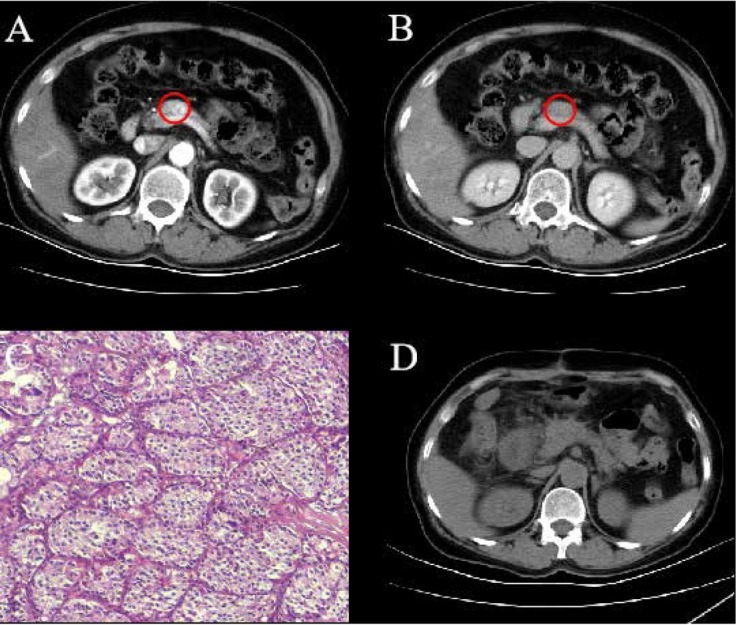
A 65-year-old female with insulinoma in the neck of the pancreas was treated with laparoscopic RFA. (A) Contrast-enhanced CT scan depicted a markedly enhanced lesion in the neck of the pancreas in the early arterial phase (red cycle). (B) Contrast-enhanced CT scan showed an isointense lesion in the delayed phase (red cycle). (C) Pathology confirmed the diagnosis of pancreatic insulinoma (HE×100). (D) The postoperative CT scan suggested the tumor was completely ablated.
